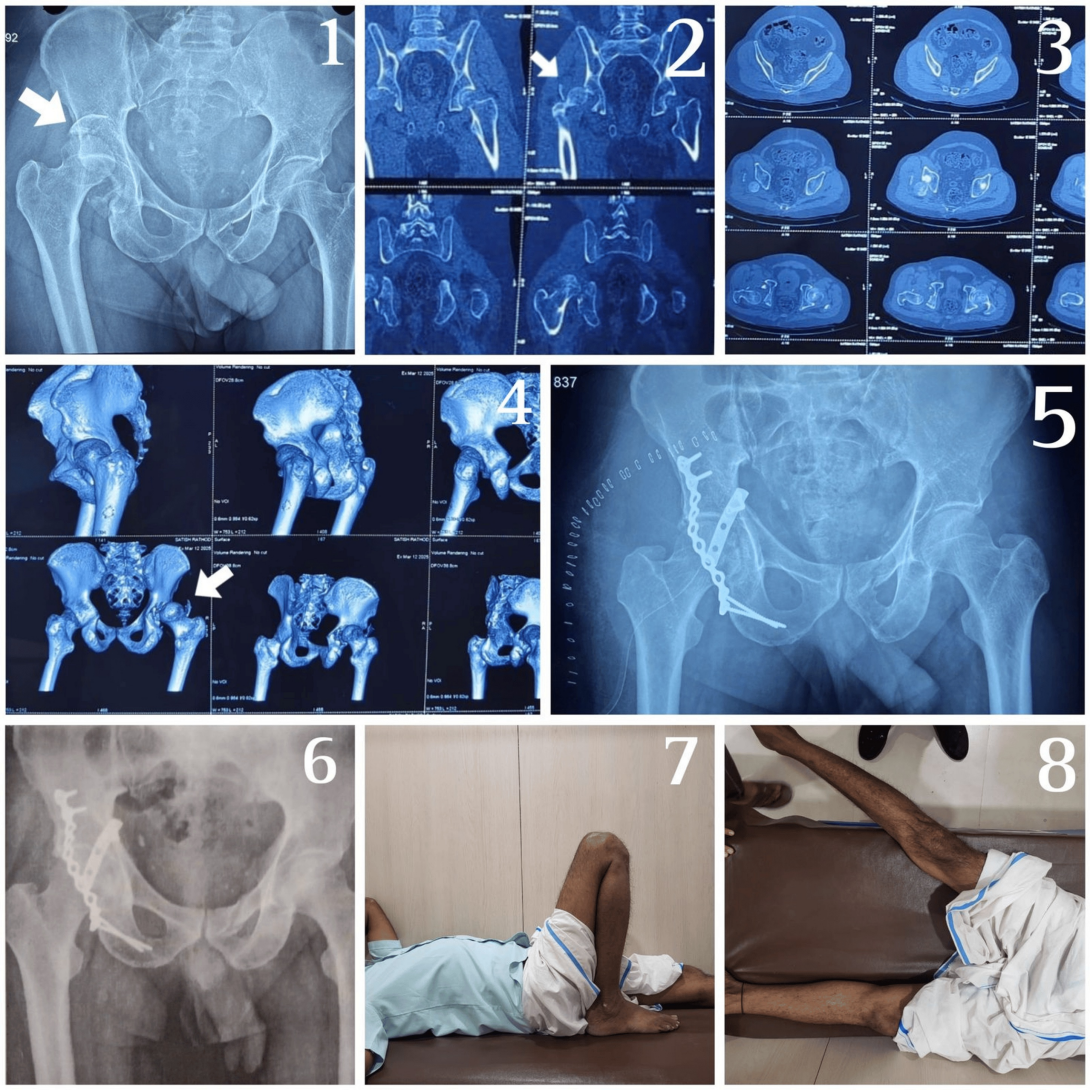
Pre- and postoperative radiographs of a patient with a posterior column fracture using the Kocher-Langenbeck approach First: Preoperative X-ray of the pelvis with both hip joints showing posterior hip dislocation with posterior acetabular wall fracture, which was reduced using the modified Allis method; second, third, and fourth: Computed tomography (CT) images—coronal, axial, and 3D reconstruction cuts, respectively, with fracture fragment reduced; fifth: Immediate postoperative X-ray of the pelvis with both hip joints; sixth: Postoperative X-ray at six-month follow-up; seventh and eighth: Hip movements postoperatively at six months showing excellent results.
